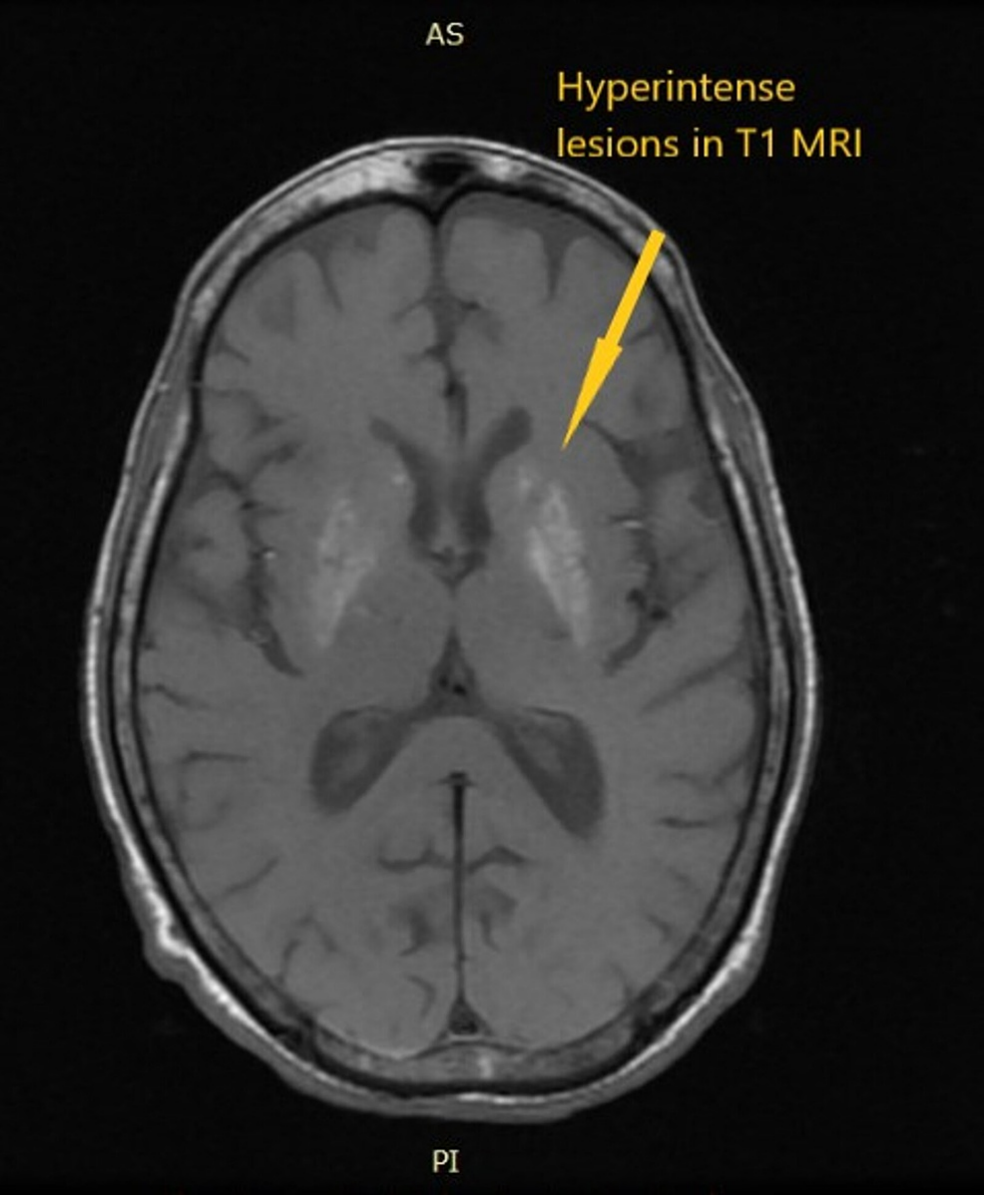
Hyperintense lesions in bilateral basal ganglia in T1 MRI.
